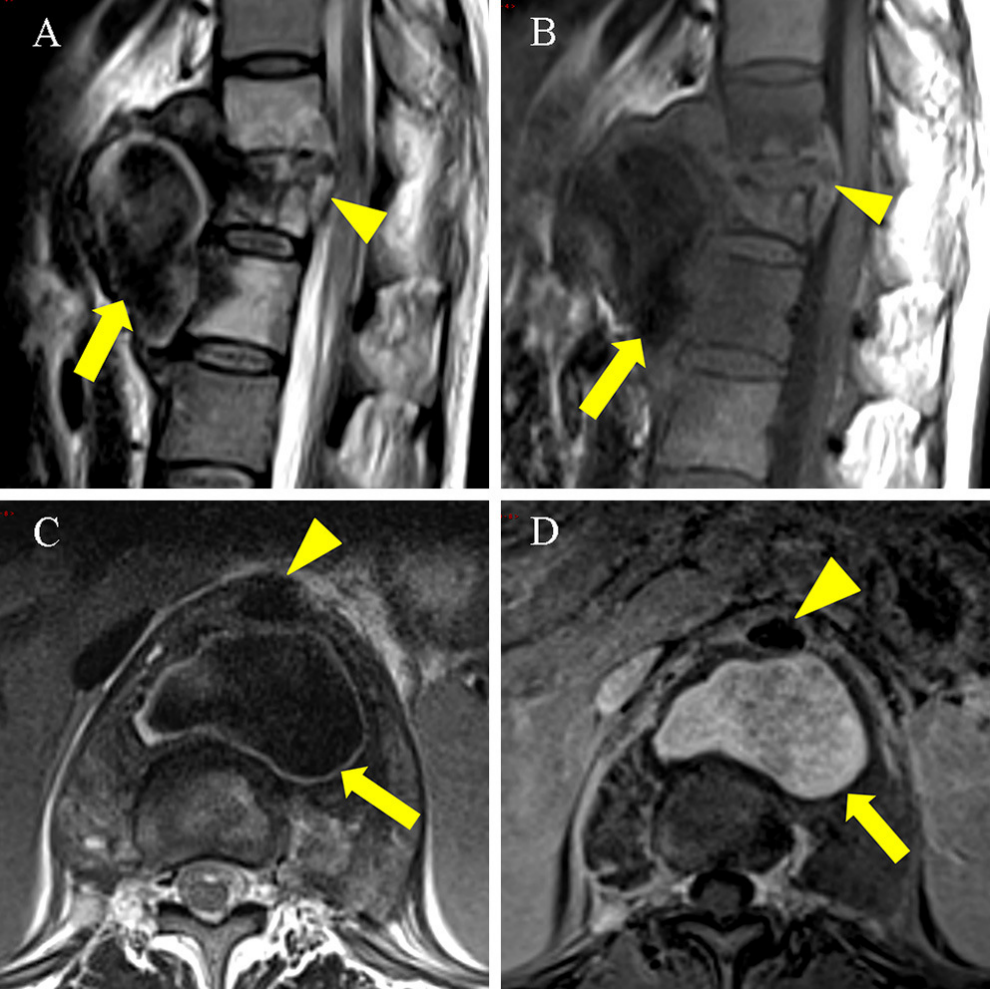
An enhanced MRI scan on 6 November 2021 showed a pseudoaneurysm measuring 5.7 × 3.7 × 5.8 cm. (A) (T2WI), (B) (T1WI): Sagittal MRI scan showed T11-L1 with bone destruction (arrowhead). Large cold abscess in the front of the vertebral body with flow void signal (thick arrow). (C) T2WI axial MRI scan showed a cold abscess with flow void signal (thick arrow) located posterior to the thoracic aorta (arrowhead). (D) Axial enhanced MRI scan showed the pseudoaneurysm (thick arrow) located posterior to the thoracic aorta (arrowhead) was hyperintense.
